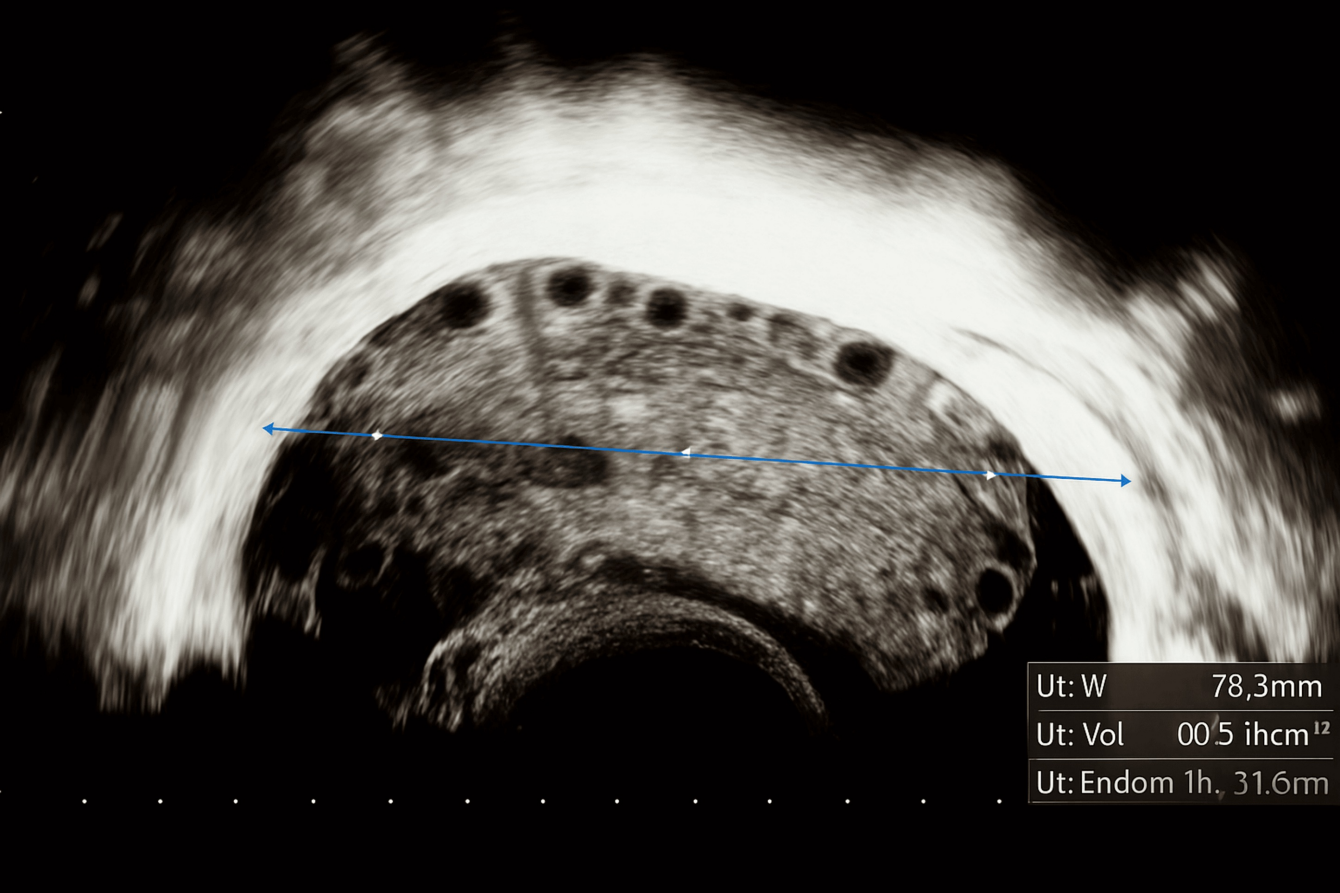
TVUS showing an enlarged right ovary (approximately 7-8 cm, arrow) with peripheral follicles and increased stromal echogenicity. TVUS, Transvaginal ultrasound
